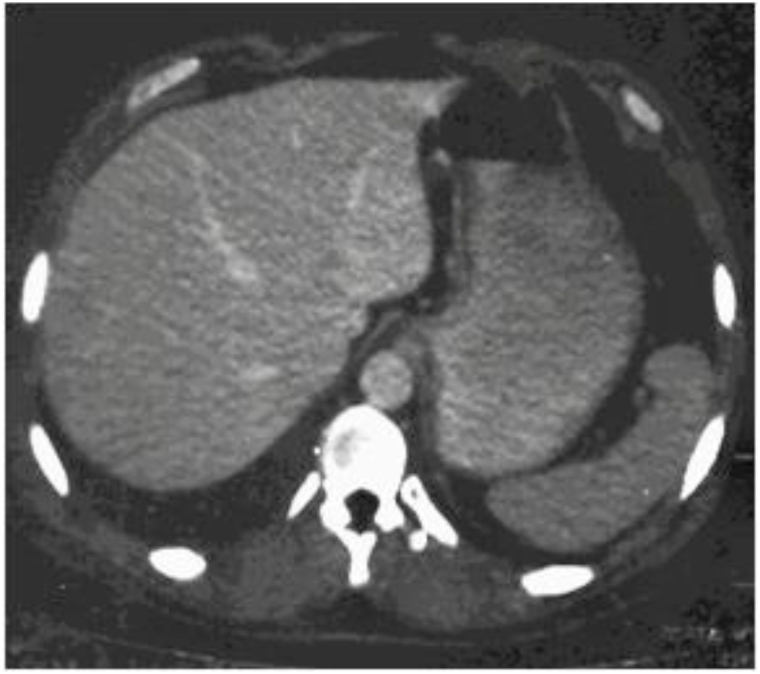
CT scan of the postoperative period. Absence of focal or cystic formations in the liver.
